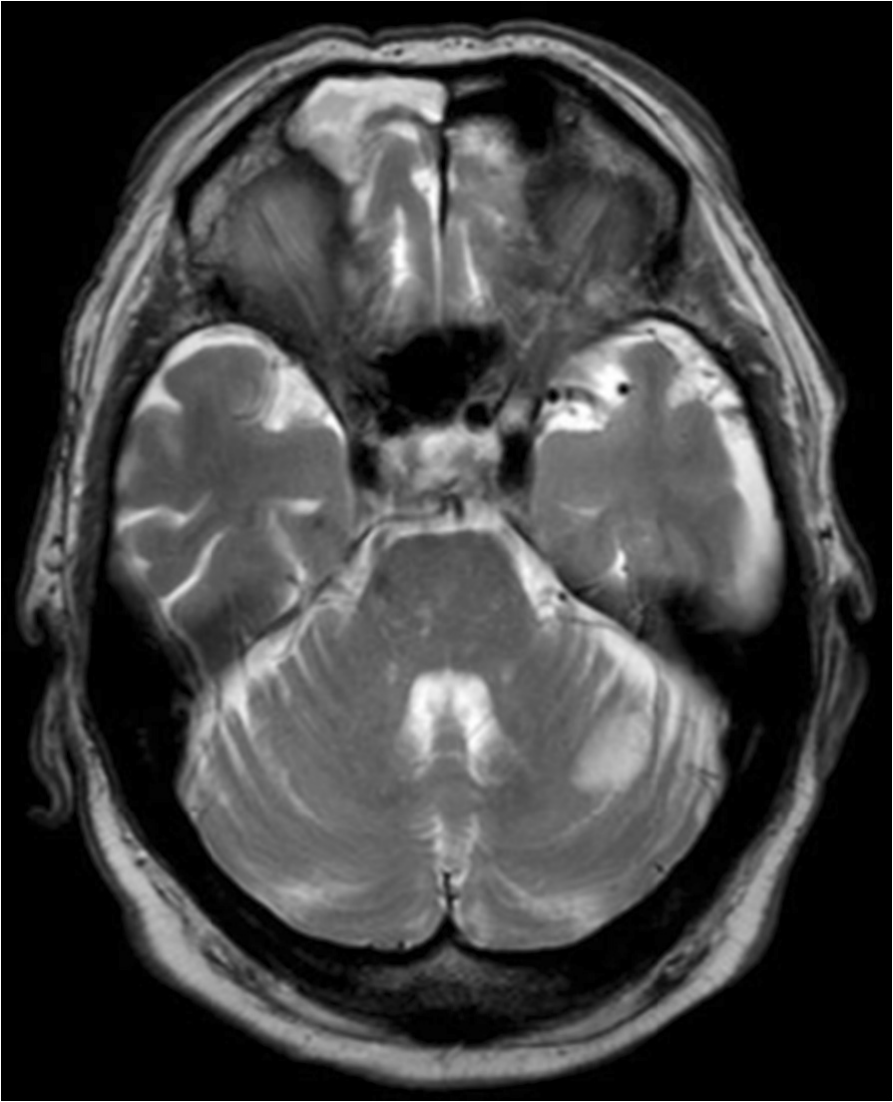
T2-weighted MRI showing the infarction in the left cerebellar hemisphere.
